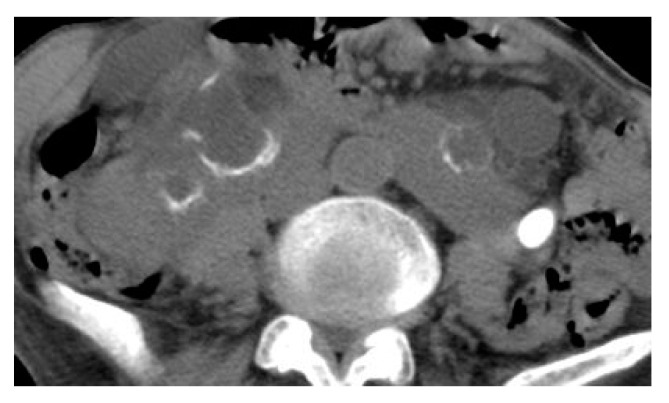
Encrusted pyelitis in horseshoe kidney. Axial non-contrast CT image shows horseshoe kidney with malrotation of both compartments and marginally calcified calyces.
